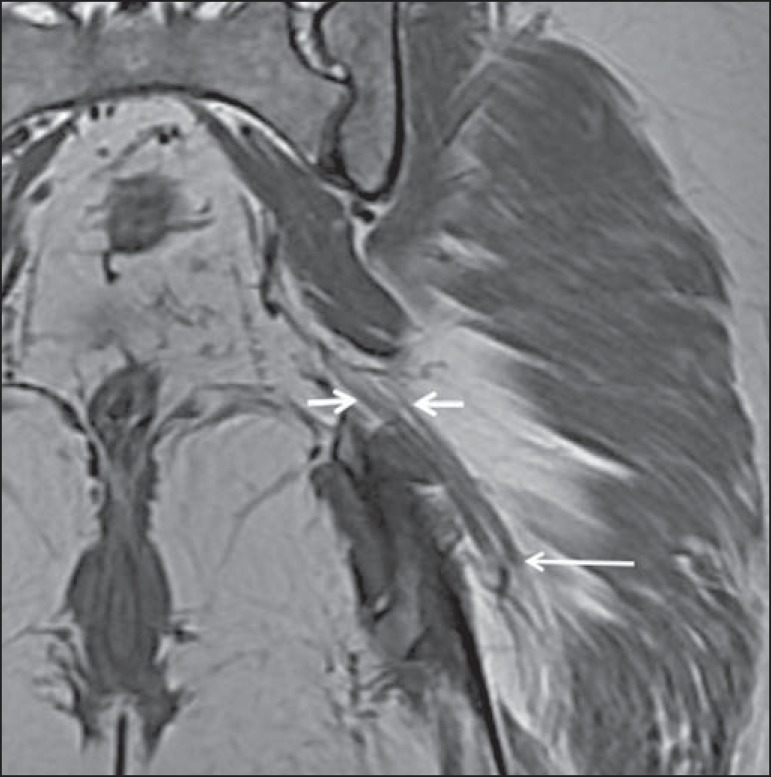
Coronal T1-weighted MRI scan showing the fascicular pattern (long arrow)
